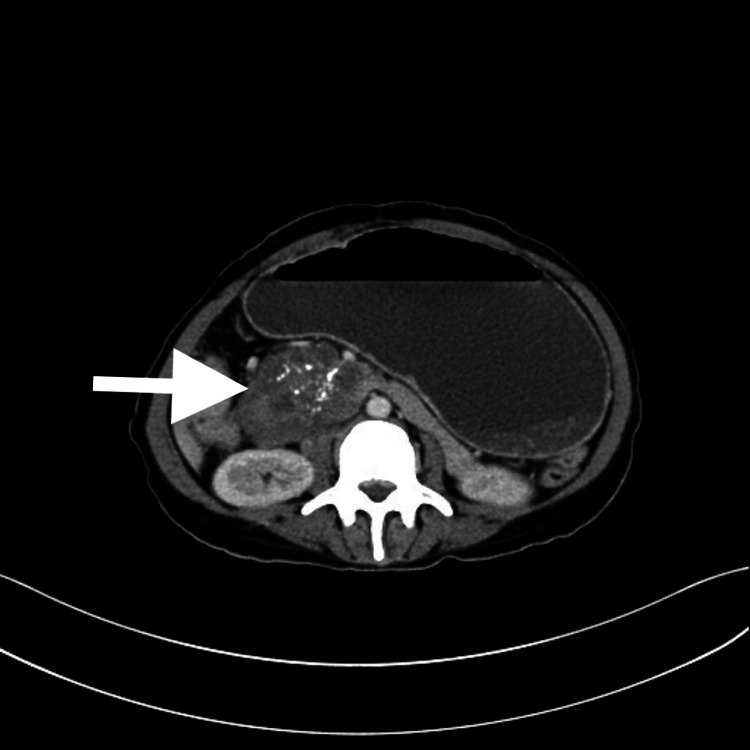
Axial image of the abdomen CT scan showing a mass in the head of the pancreas (white arrow)
